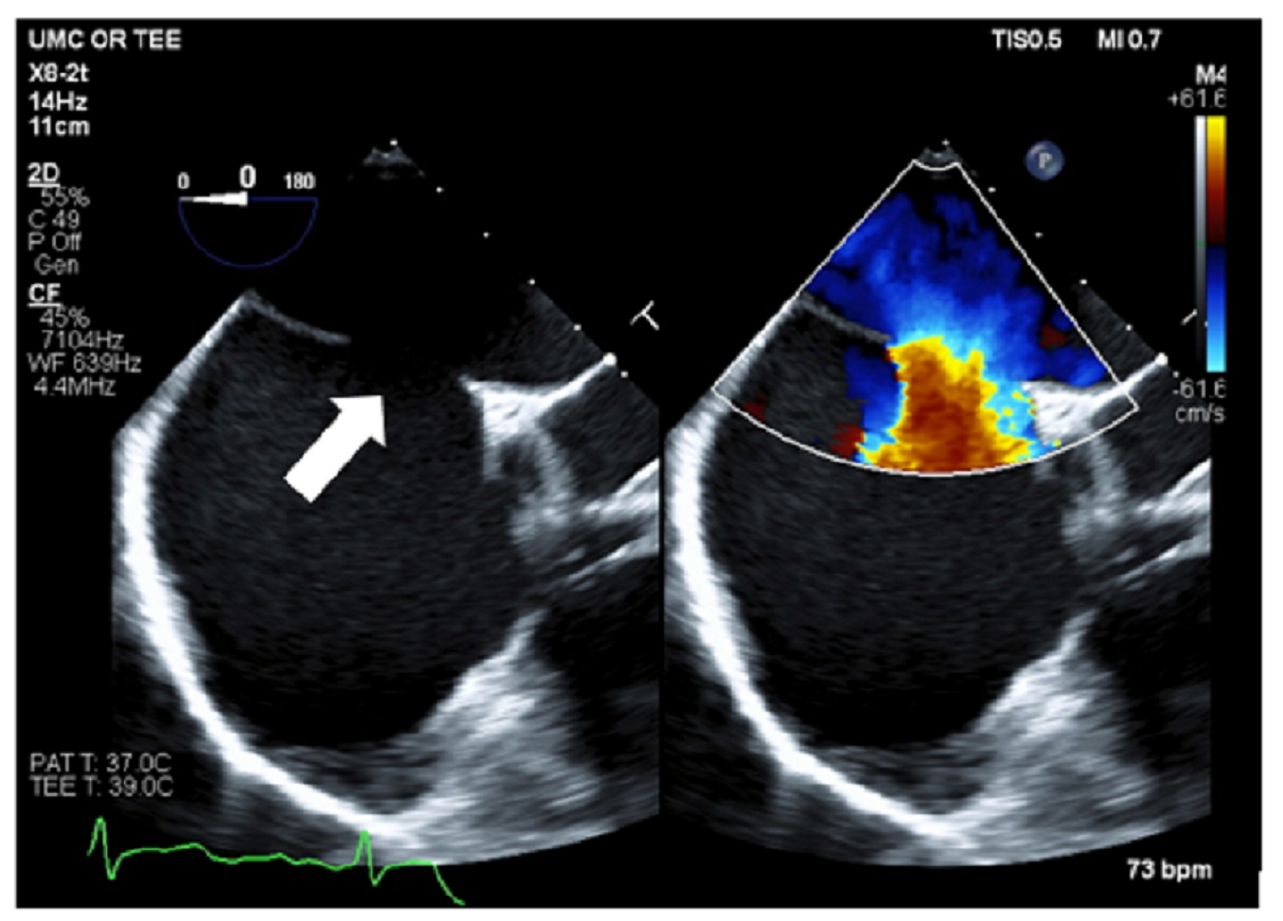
Mid-esophageal 4 chamber view showing the large ASD (white arrow) with left to right shunting using color flow Doppler. ASD: atrial septal defect
